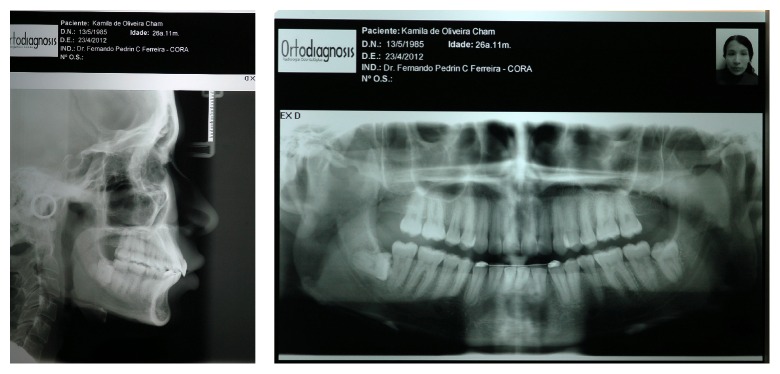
Final panoramic radiography and teleradiography.
